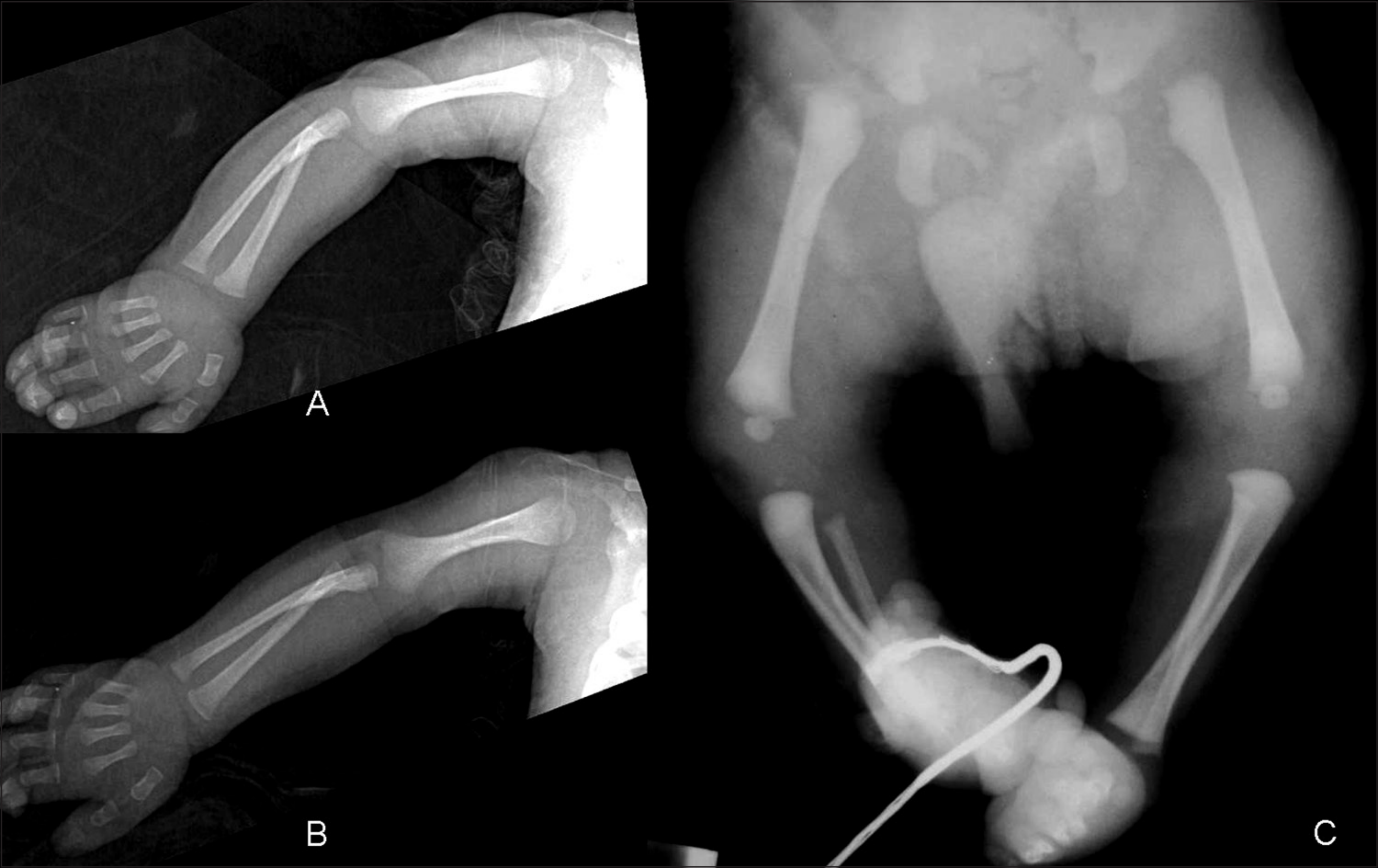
(A–C)Arthrogryposis: Frontal (A) and lateral (B) radiographs of the elbow show extended elbows, pronated forearms, and flexed wrists and fingers in a baby with arthrogryposis. Frontal radiograph of the lower limbs (C) of another baby with arthrogryposis shows bilateral hip dislocations and club feet
